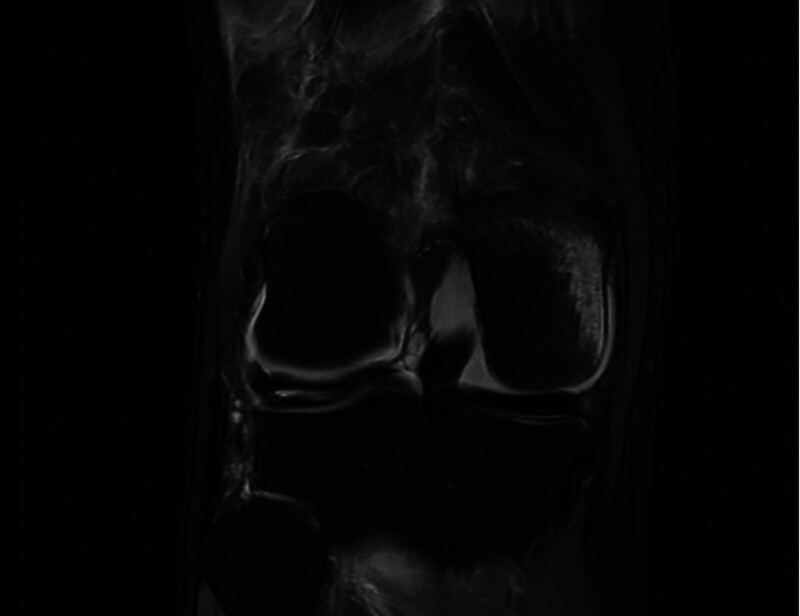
Coronal view: T1-weighted coronal images showing large fat-signal mass in the rear aspect of the distal femur.
